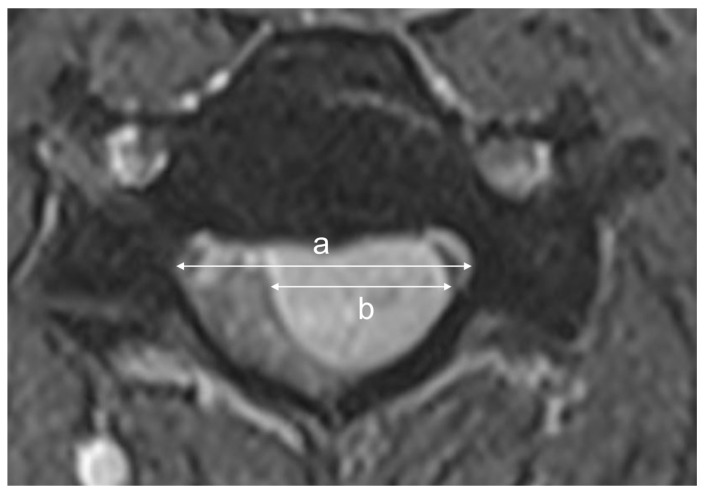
Measurement method for the Tumor-Canal Invasion Ratio (TCR). On the axial magnetic resonance imaging (MRI) slice showing the maximal tumor dimension, the spinal canal width (a) is defined as the interpedicular distance. The maximal tumor width (b) is measured at the same level. The TCR is calculated using the formula: TCR = (b/a) × 100 (%).
